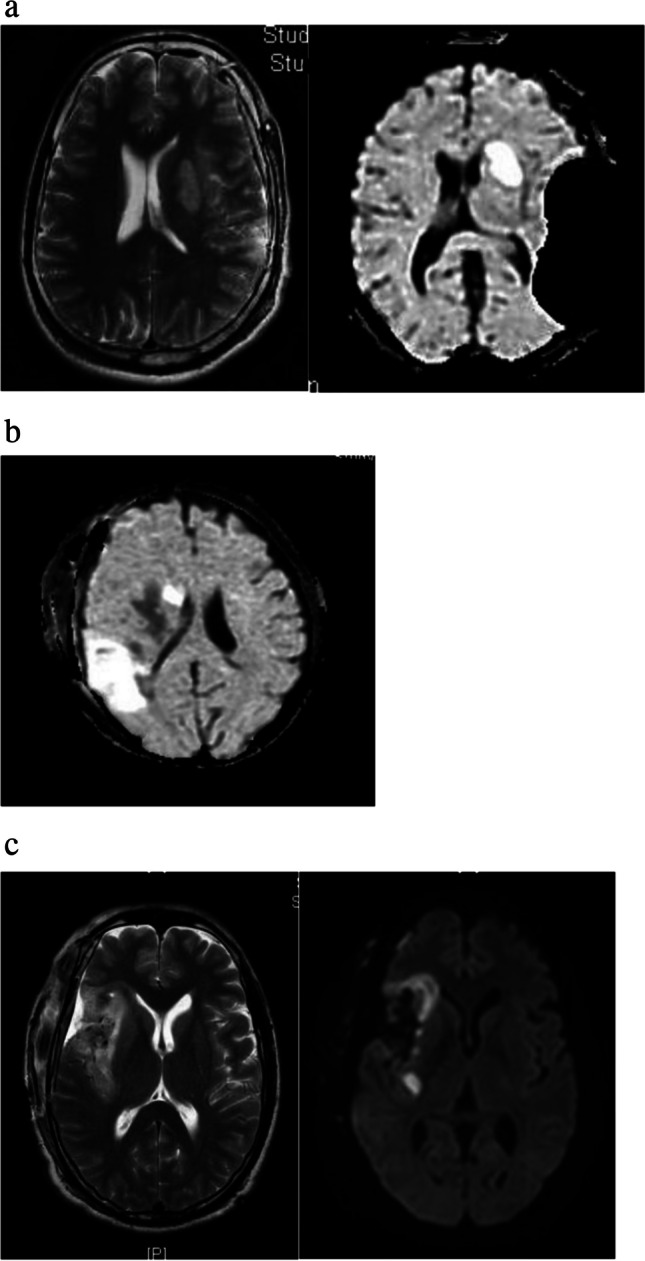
Different types of arterial infarcts on postoperartive MRI- a LSA infarct on T2W MRI and DWI, b DWI showing a cortical and deep perforator infarct c Peri-resection venous infarct on T2W MRI and DWI MRI
